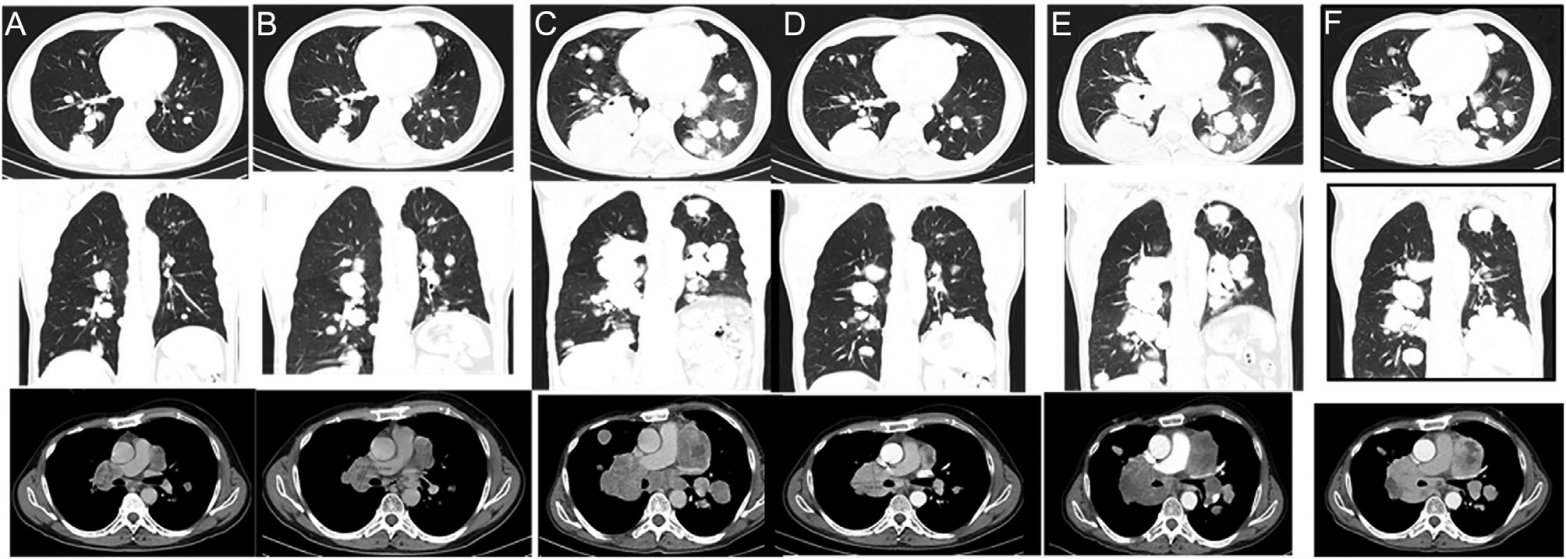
CT findings in the chest during treatment. Evaluation after sorafenib (A), docetaxel/doxorubicin (B), sunitinib (C), 12 cycles with GEMOX (D), lenvantinib (E), and 19 additional cycles of GEMOX (F).
